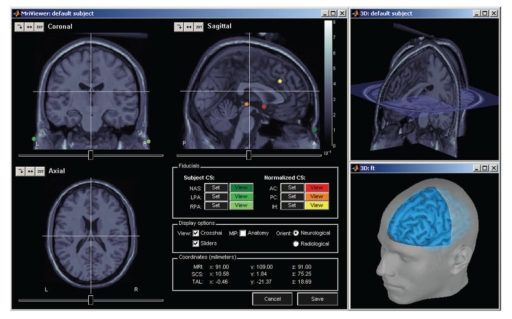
MRI and surface visualization.
